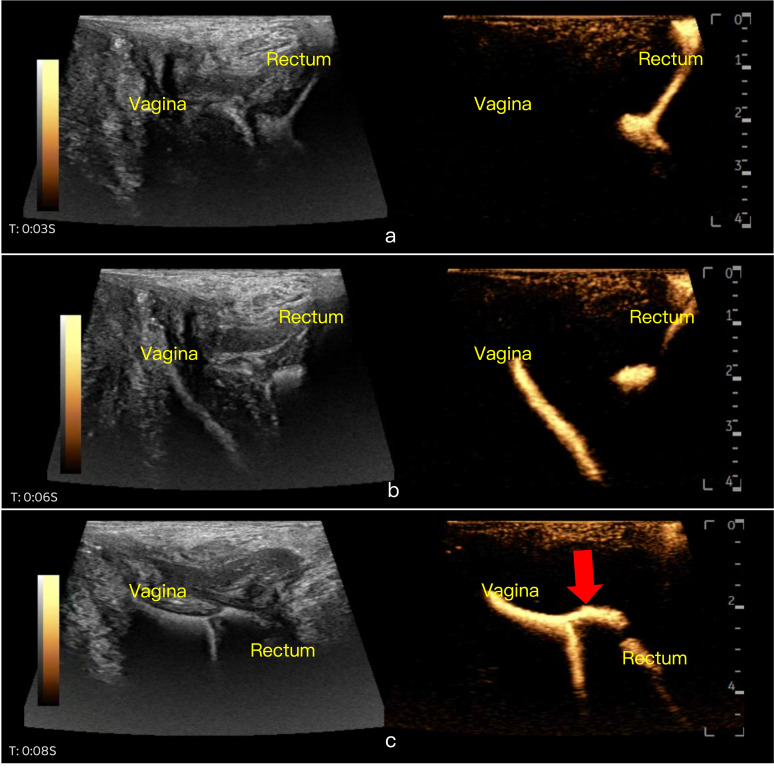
Real-time dynamic observation during contrast-enhanced ultrasound enema. (a) At T: 0:03 s, mid-sagittal TPUS image showing contrast delineating the rectal lumen. (b) At T: 0:06 s, contrast agent entering the vaginal cavity. (c) At T: 0:08 s, after probe angle adjustment, thecontrast agent delineates the course of the rectovaginal fistula (indicated by the red arrow).
